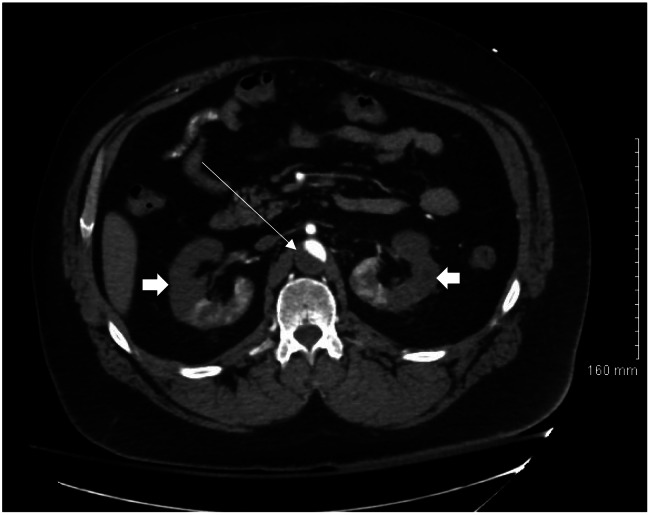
Computed tomography angiogram abdomen in axial plane showing aortic thrombus (thin arrow) and renal cortical hypodensities (thick arrows).
